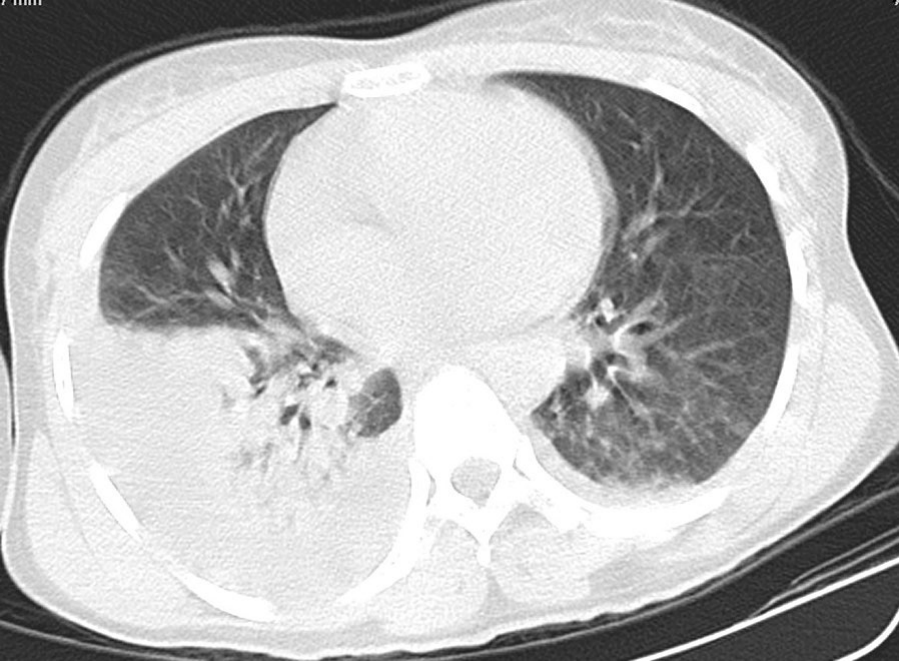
Twenty-six years old female patient with bilateral pleural effusion and consolidation with air bronchograms at the right lower lobe are seen in CT scanning of the chest
